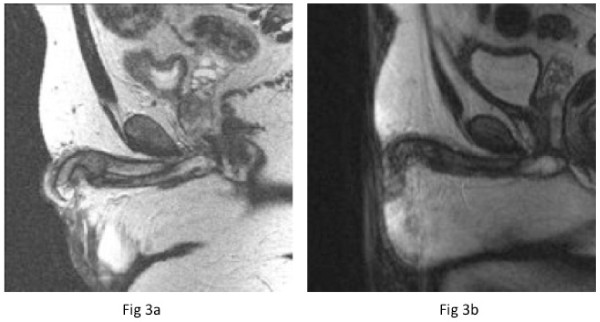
MRI Scans: (a) Pre-chemotherapy T2 sagittal thin penile MRI slices demonstrating the penile metastasis within the corpora. (b) Post-chemotherapy T2 sagittal thin penile MRI slices showing the resolving penile lesion.
